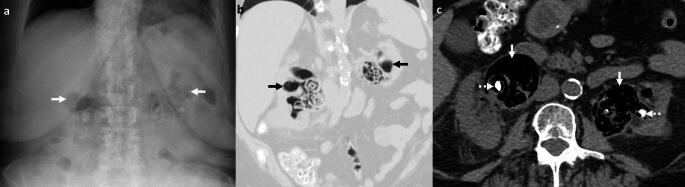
Emphysematous Pyelitis. A 42-year-old with renal calculi, dysuria, and no prior intervention. Abdominal radiograph (a) shows air in bilateral pelvicalyceal systems outlining renal calculi (white arrows, a). CT images show air localized to bilateral renal collecting systems and pelvis (black solid arrows, b, and white solid arrows, c) and outlining renal calculi (dotted white arrow, c). Note the absence of air in the renal parenchyma, excluding emphysematous pyelonephritis
